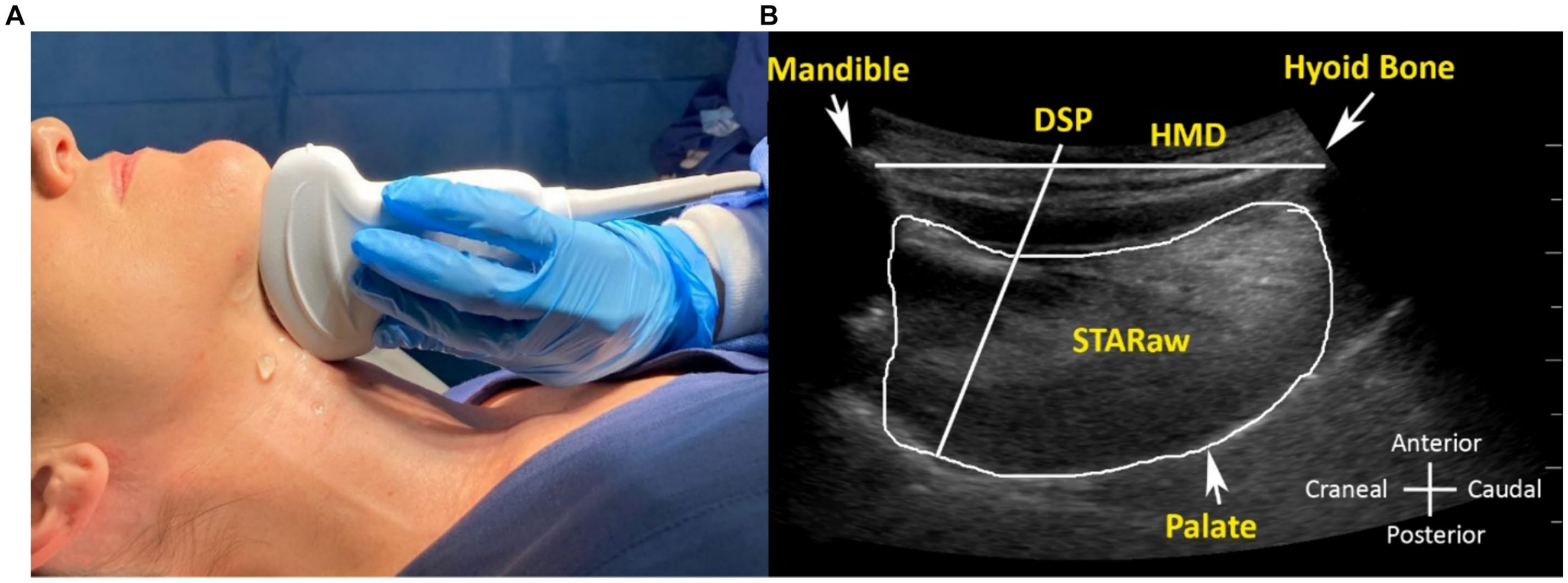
(A,B) Ultrasound image measurements in awake patient with the corresponding probe position. (B) Hyomental distance (HMD), sagittal tongue area in awake patient (STARaw) and distance from skin to the deepest part of palate (DSP).
